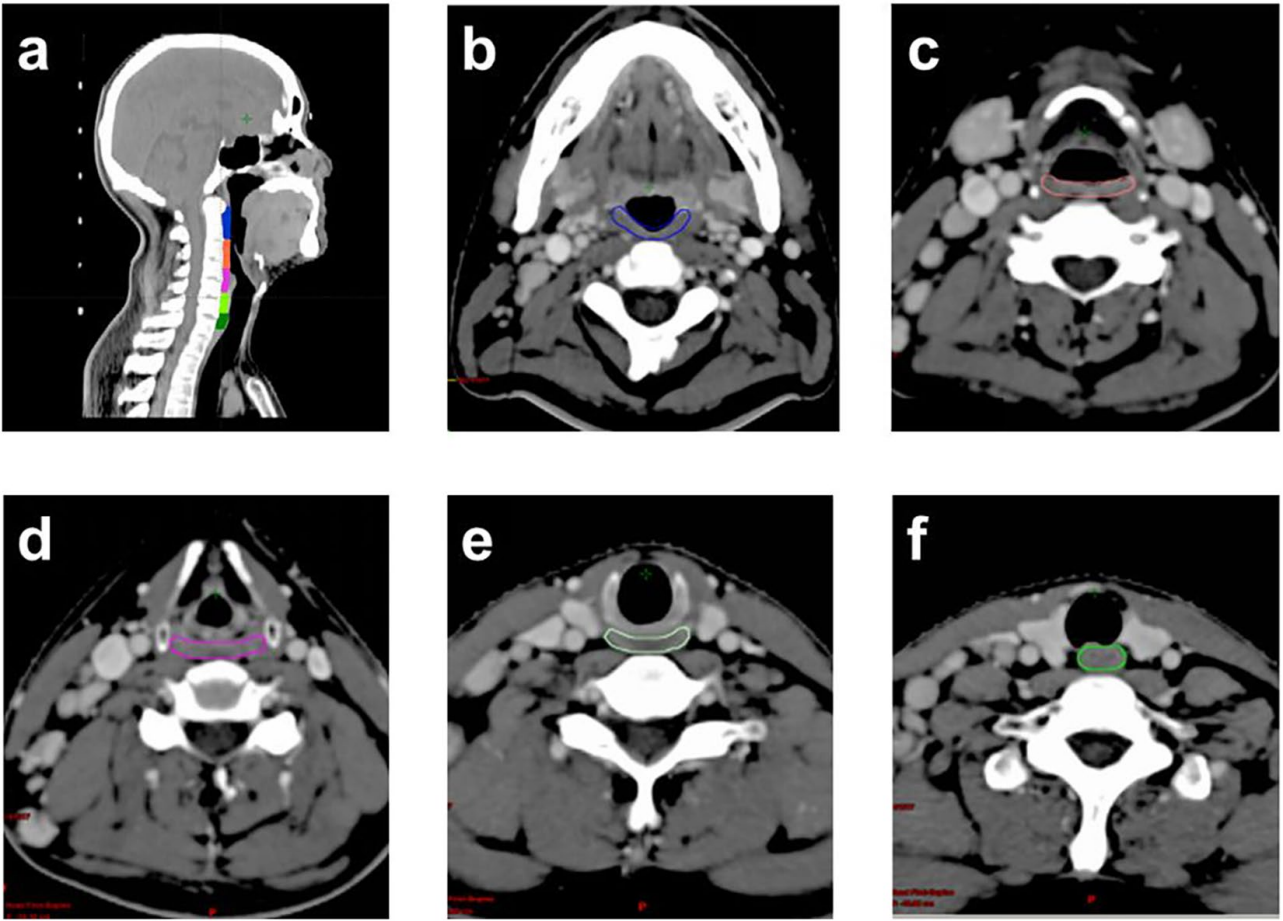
Anatomical delineations of the SWOARs. (a) Delineations on sagittal CT-slices. (b–f) The contours of the SCM, MCM, ICM, CPM and EIM on axial CT-slices.
